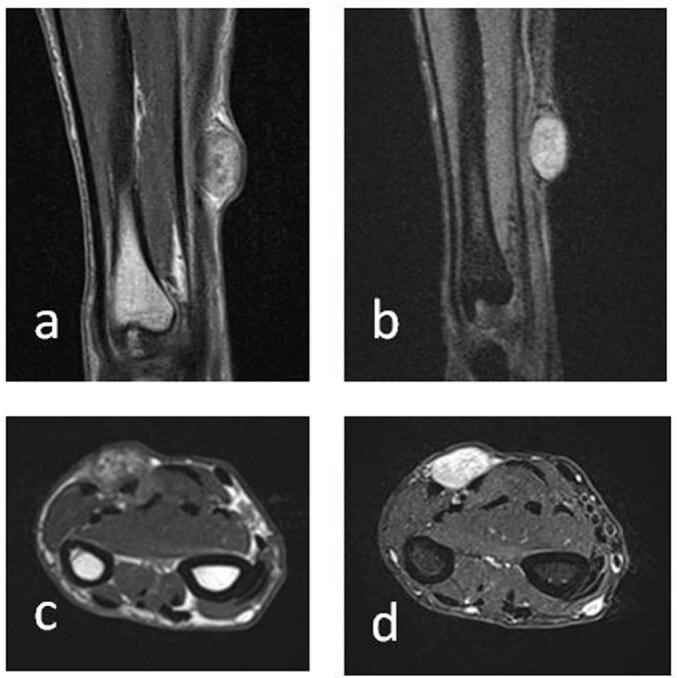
MRI of the forearm showing the lesion. A) T2-weighted coronal section B) Gadolinium-enhanced T1-weighted coronal section C) T2-weighted axial section D) Gadolinium-enhanced T1-weighted axial section.
